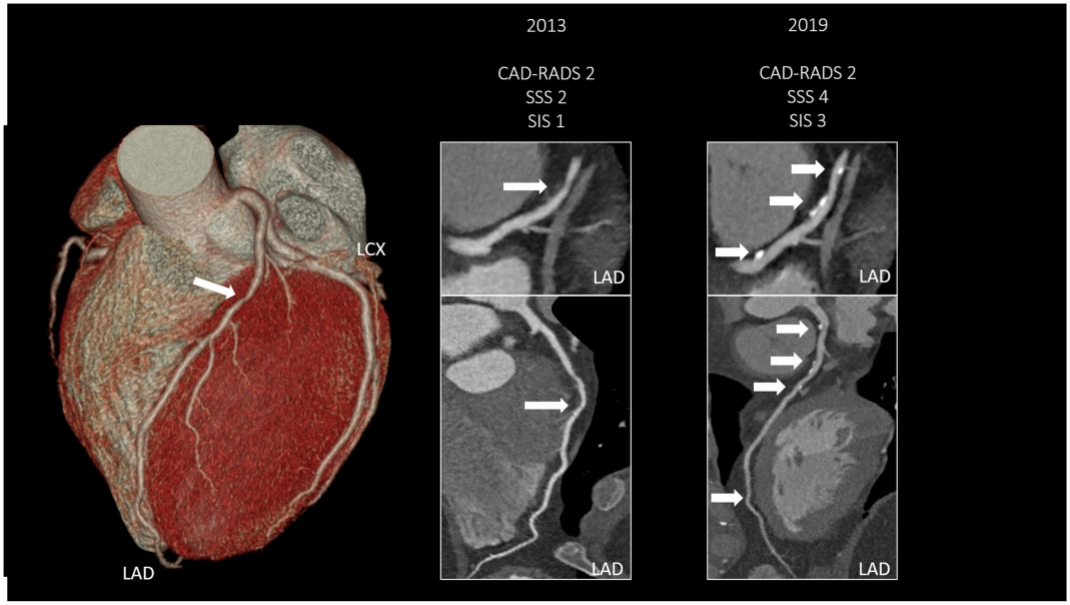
Representative case of plaque progression. A 51-year-old male patient underwent serial CTA scanning due to stable chest pain. In 2013, the patient had one affected coronary segment (mid-LAD) with plaque. The figure shows the LAD with a non-calcified plaque leading to mild stenosis (CAD-RADS 2, SSS 2, SIS 1). We detected coronary plaque progression on the proximal and distal LAD segment of LAD 6 years later, including *de novo* calcified plaque development. The total segment involvement and segment stenosis scores were both higher on follow-up (SSS 4 and SIS 3), however, based on the worst stenosis CAD-RADS score of 2 was assigned. CAD-RADS, Coronary Artery Disease—Reporting and Data System; LAD, left anterior descending artery; LCX, Left circumflex artery; SIS, segment involvement score; SSS, segment stenosis score.
